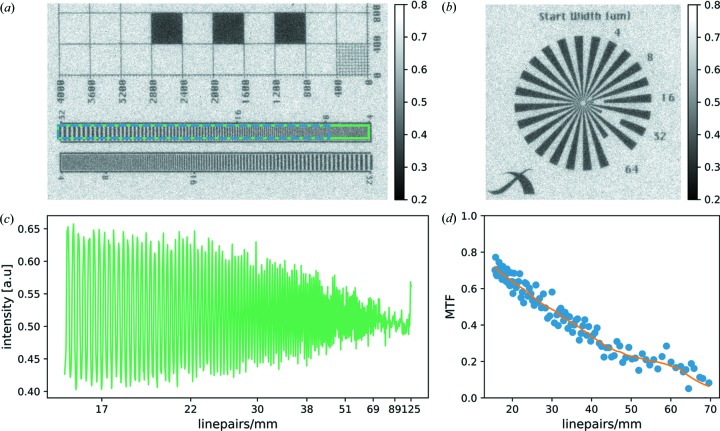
Analysis of the resolution of the XBM. (*a*) The line pattern recorded for determination of the X-ray camera resolution. The Siemens star (*b*) demonstrates isotropic resolution of the camera. (*c*) The intensity modulation of the line pattern indicated with a green box in (*a*) averaged along the lines. (*d*) The MTF values calculated from the raw data depicted in (*c*). The orange line is a moving average (Savitzky–Golay) of the MTF values. At 65 line-pairs mm^−1^ the MTF is 0.1 which fulfills the requirement of resolving the blur caused by a 50 µm X-ray source.
